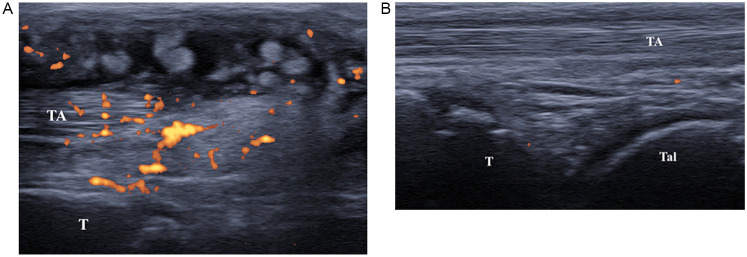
(A) Ultrasound image (longitudinal scan) of anterior tibial tendon. Presence of anechoic peritendinous effusion and severe synovial hypertrophy with hyperechoic synovial villi and diffuse power Doppler signal. (TA, tibialis anterior; T, tibia). (B) Ultrasound image (longitudinal scan) of anterior tibial tendon. Presence of minimal anechoic peritendinous effusion and minimal synovial hypertrophy. (TA, tibialis anterior; T, tibia; Tal, talus).
